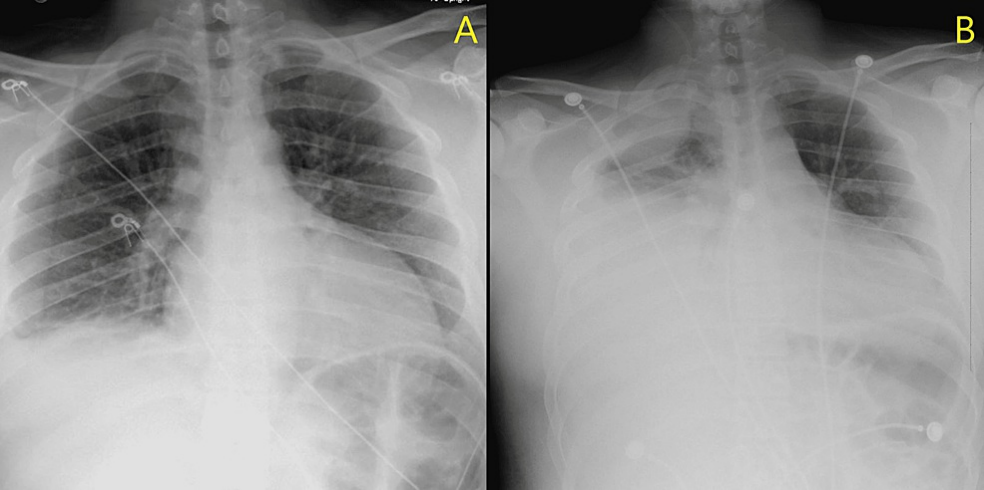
Chest x-ray images demonstrating rapid accumulation of a loculated pleural effusion. (A) Initial presentation and (B) three days later.
